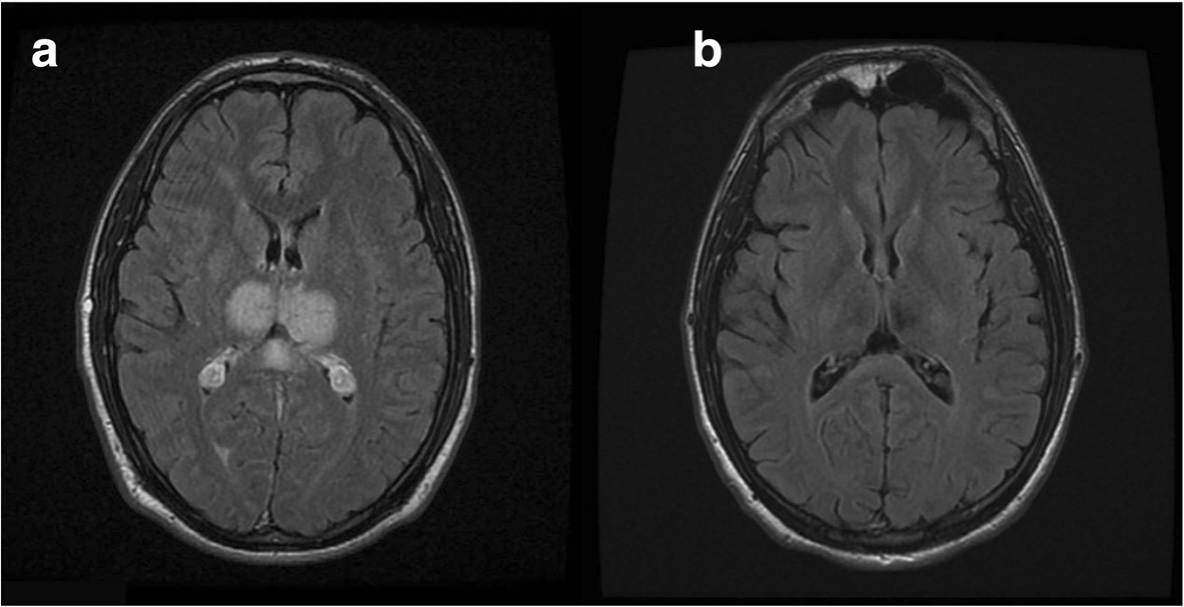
Fluid attenuated inversion recovery axial magnetic resonance imaging showing bithalamic hyperintensities (a), reversible with anticoagulation therapy (b).
